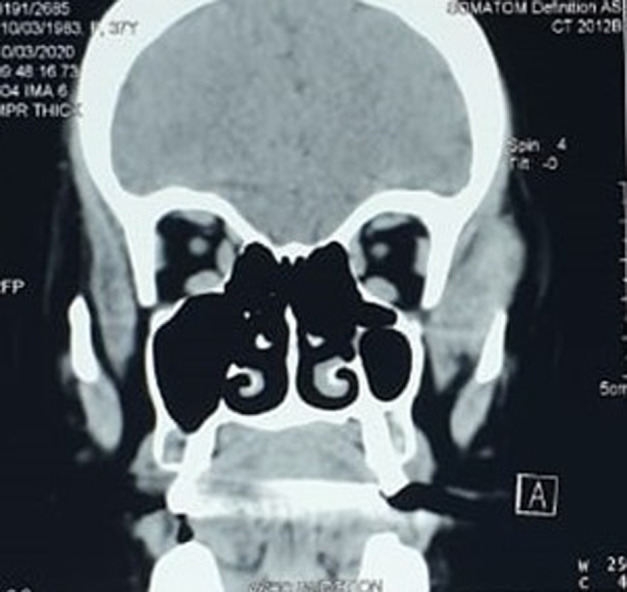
computed tomography scan on the coronal plane shows the mass in the left temporal region with no erosion of the bone
